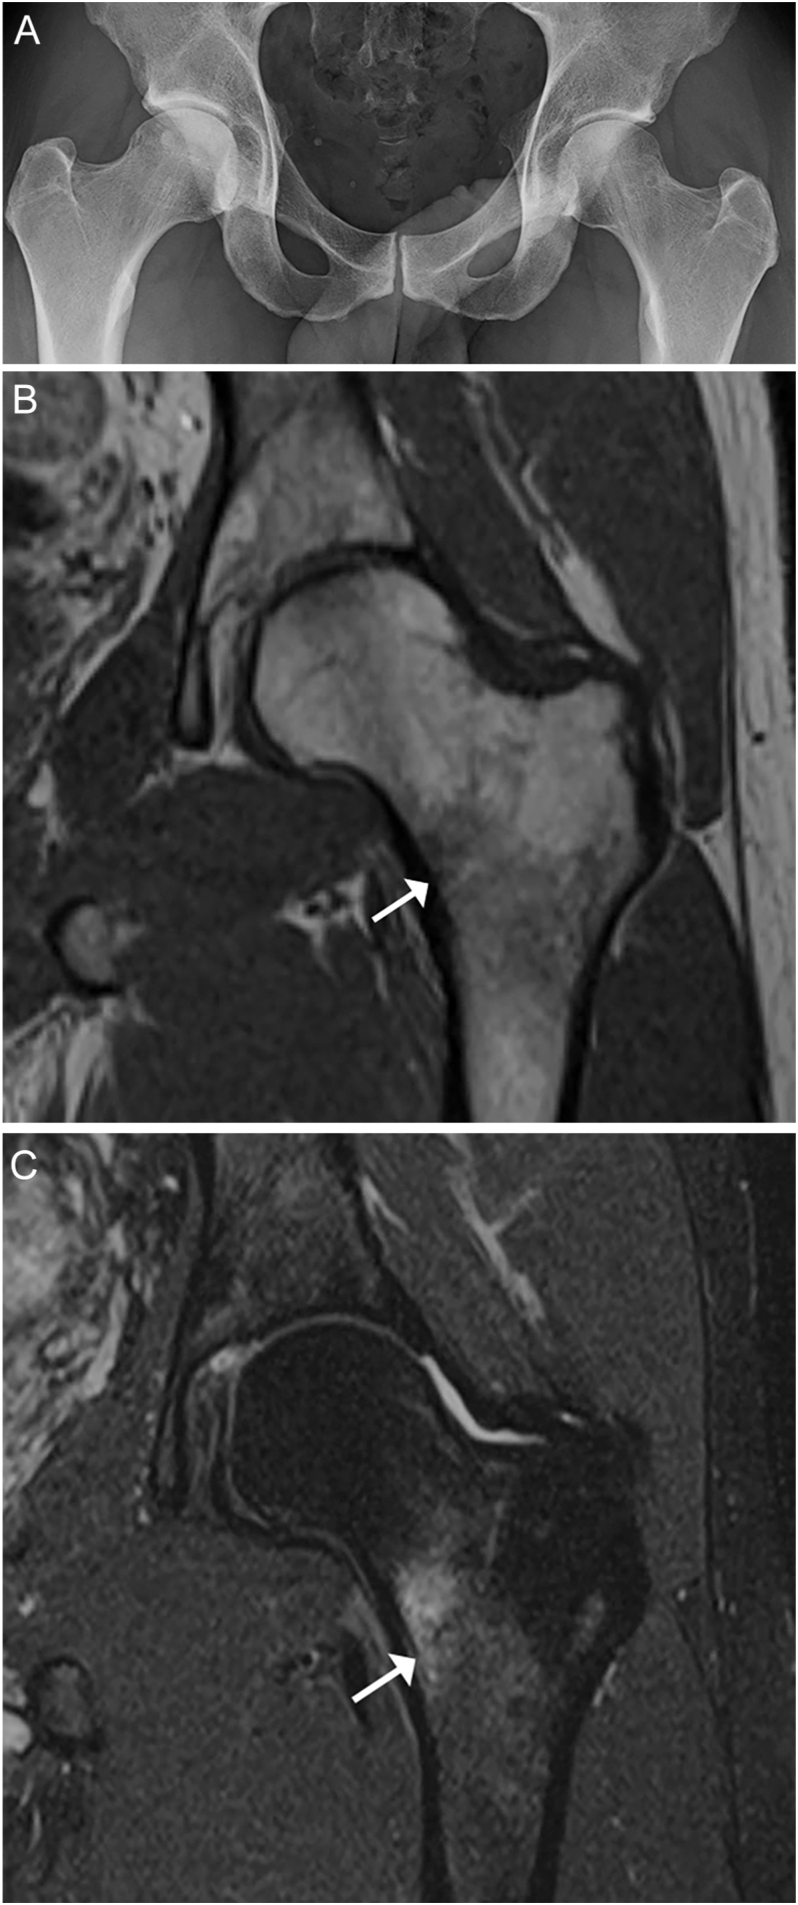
A 35-year-old male with recurrent left hip pain – femoral neck stress fracture. AP radiograph of the pelvis (A) demonstrates a normal appearance of the hips. Coronal T1 (B) and T2 fat-suppressed (C) MR images from MRI acquired one month later demonstrate bone marrow edema, which appears hyperintense on T2 and hypointense on T1 within the medial base of the left femoral neck, and with a subtle fracture plane, which appears most conspicuous on the T2-weighted image (arrows).
